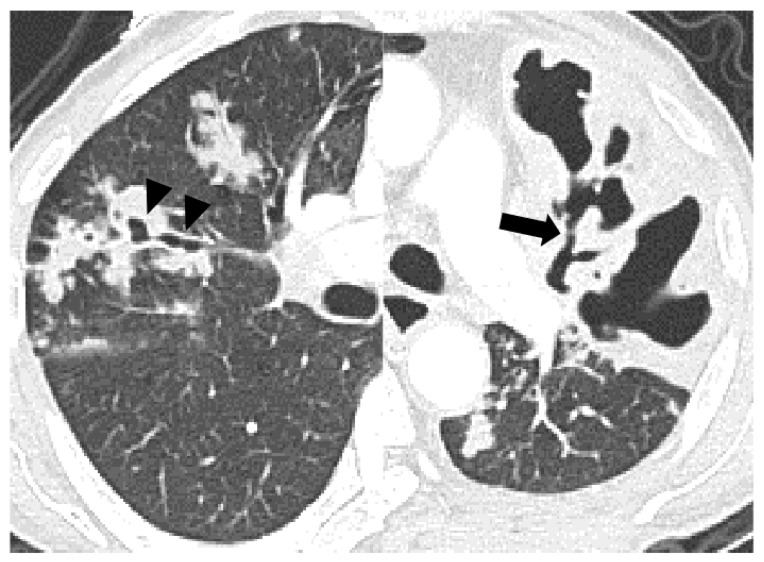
A 67-year-old male with pulmonary tuberculosis and uncontrolled diabetes (HbA1C, 9.90%). The axial CT scan shows fusiform bronchial dilatation with focal erosive or aneurysmal change (arrowheads) within the consolidation in the right upper lobe. The cavity within the consolidation in the left upper lobe communicates with the dilated anterior segmental bronchus (arrow), showing the feeding bronchus sign.
